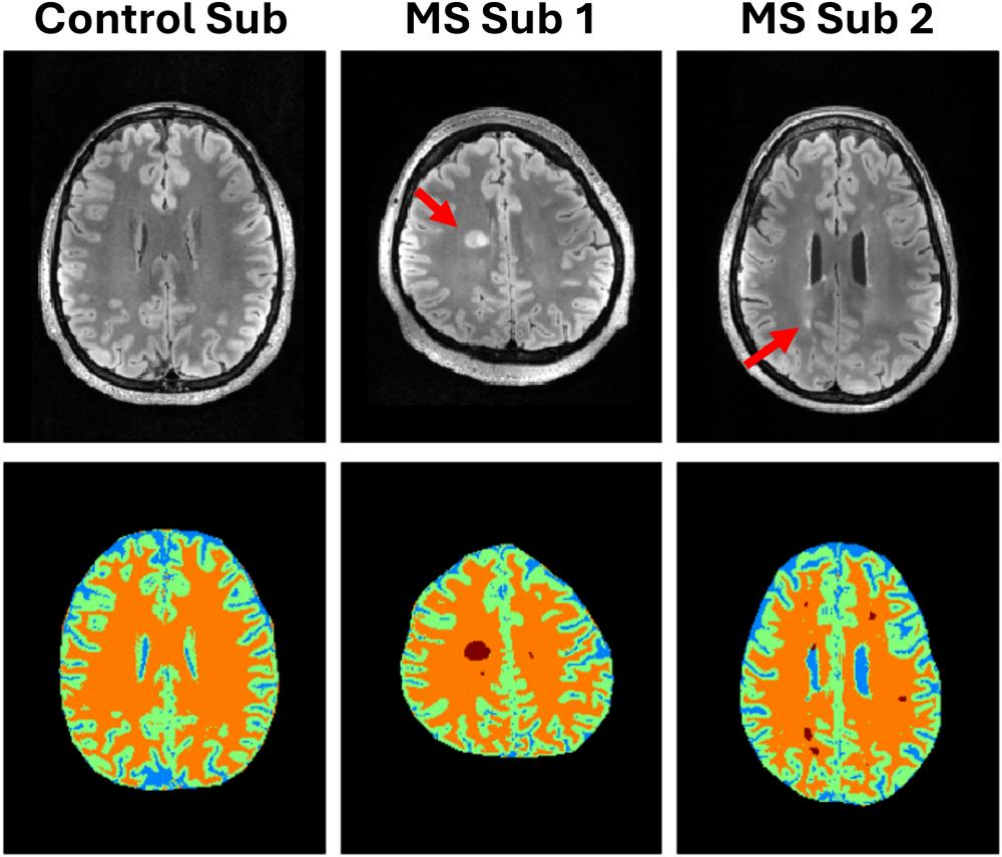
Representative structural images and segmentations. A representative set of FLAIR images (top row) for a healthy control subject and two multiple sclerosis subjects, in which prominent lesions can be seen in both subjects (red arrows). Example segmentations (bottom row) of grey matter, white matter, CSF, and identifiable lesions.
